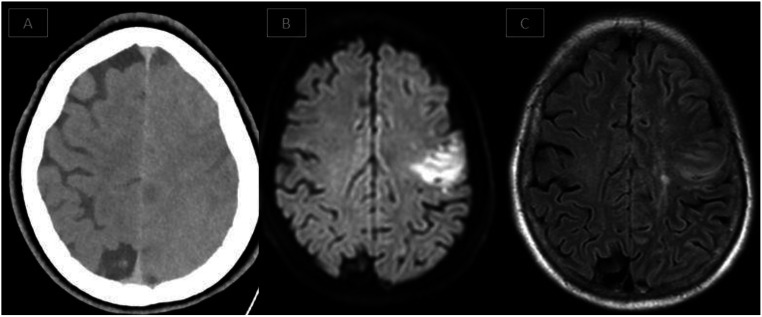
Immediate postinterventional FDCT scan and follow-up MRI of patient demonstrating reversible changes seen in contrast-induced encephalopathy: (A) cerebral edema and sulci effacement of the left hemisphere as seen on FDCT. (B) and (C) DWI and FLAIR sequences demonstrating diffusion restriction within the infarct core, along with diffuse cortical hyperintensity on FLAIR imaging extending beyond the infarcted territory, without corresponding diffusion restriction. This was accompanied by left hemispheric swelling
